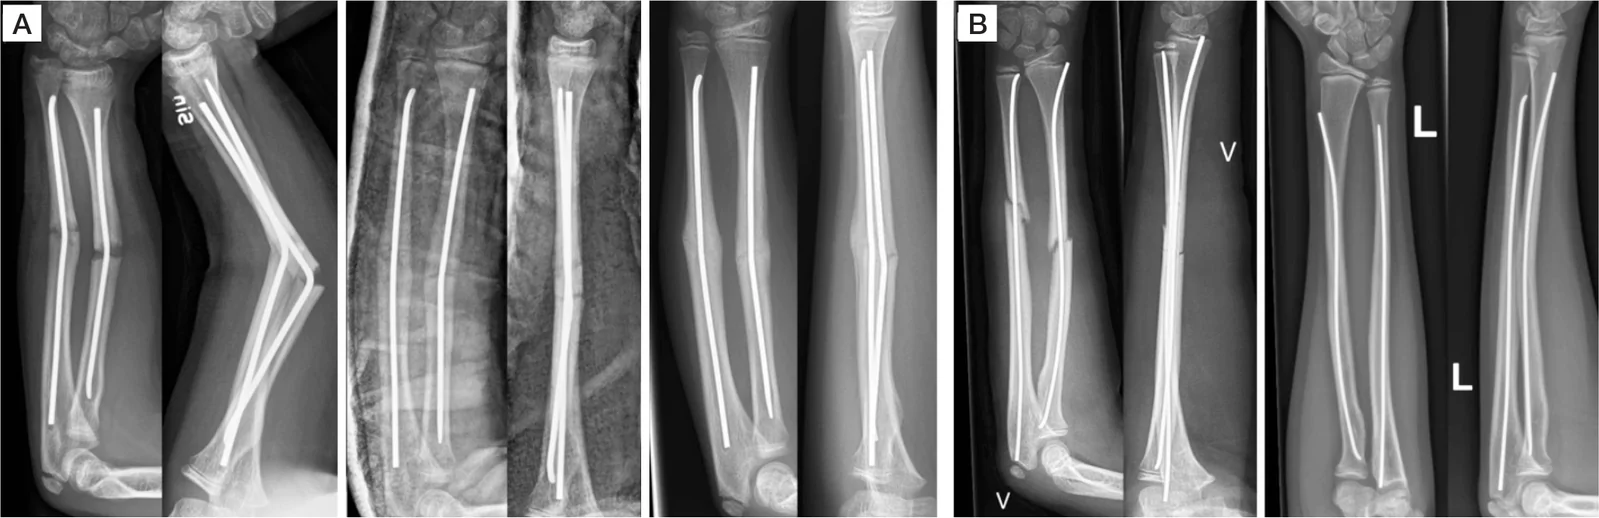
(A) Refracture with ESIN in situ 5 months after index surgery. The refracture was managed with closed reduction, with uneventful healing. The nails were not removed. (B) ESIN in situ in a 10-year-old boy. Radiographs 2 years later (right) demonstrate growth, moving the physes away from the implant. The patient remained asymptomatic, and the implants were retained.
